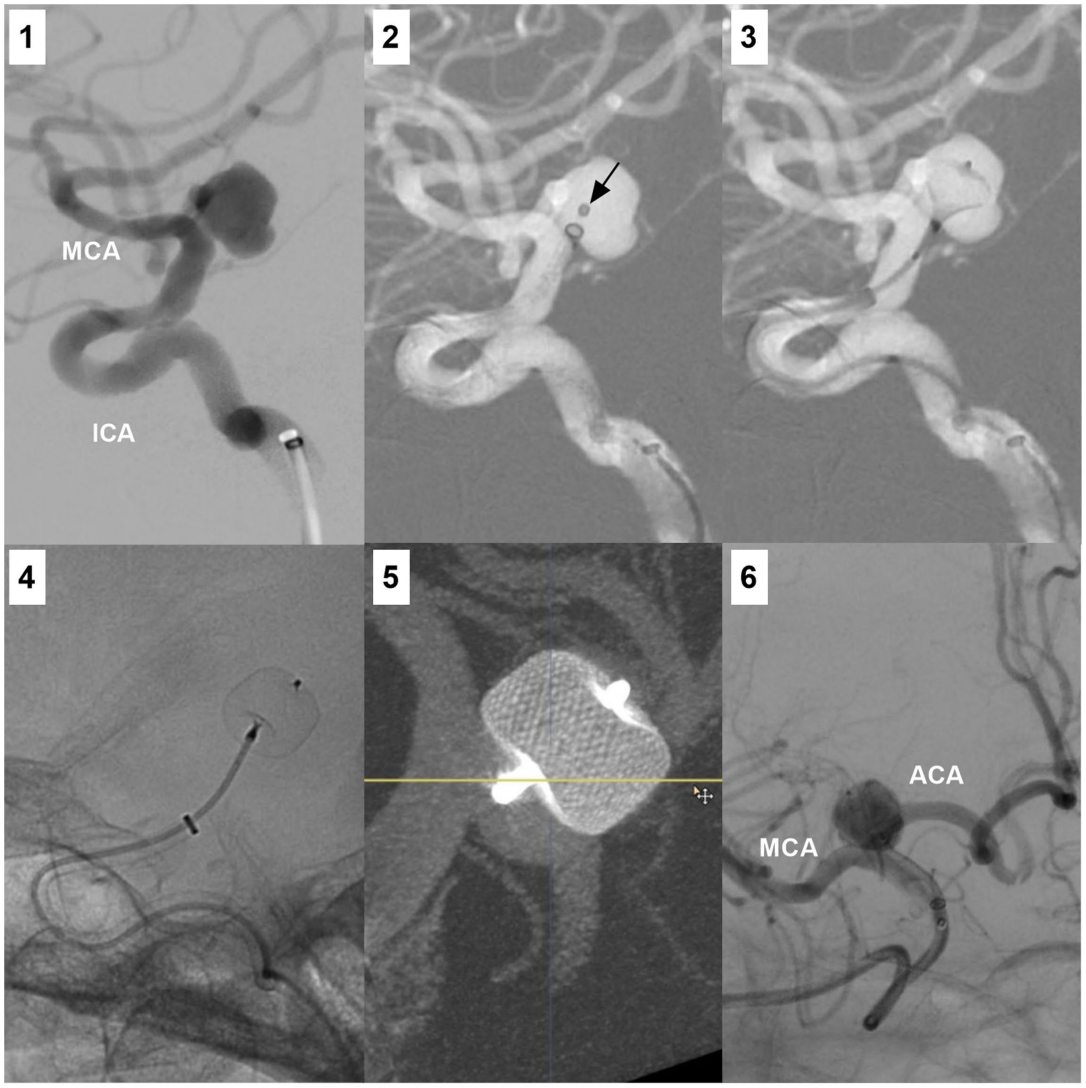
WEB device deployment for an ICA bifurcation aneurysm. Number 1 panel: DSA showing an ICA bifurcation aneurysm. Number 2 panel: Roadmap DSA showing the microcatheter (arrow) in the aneurysm. Number 3 panel: Roadmap DSA showing the partially open WEB device in the aneurysm. Number 4 panel: X-ray image showing the open WEB device. Number 5 panel: Vascular reconstructive DSA showing the WEB device in the aneurysm. Number 6 panel was the postoperative DSA. ACA, anterior cerebral artery; DSA, digital subtraction angiography; ICA, internal carotid artery; MCA, middle cerebral artery; WEB, Woven EndoBridge device.
